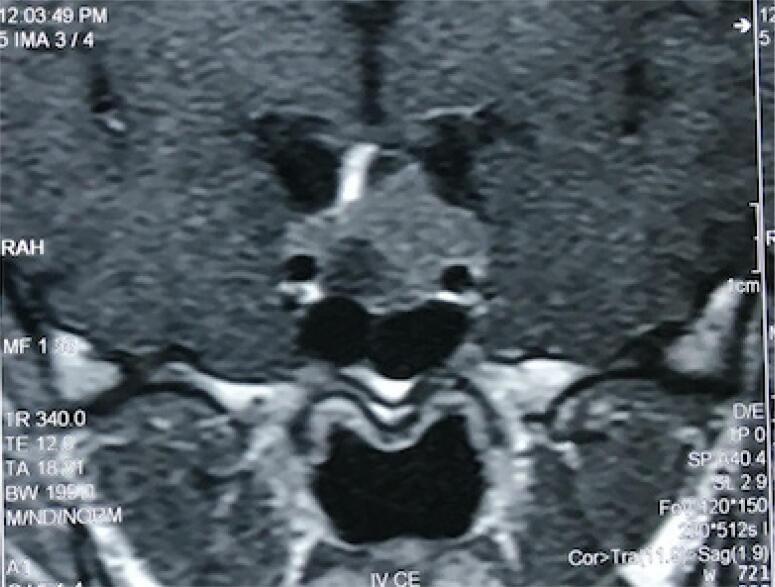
Pituitary MRI revealed a macroadenoma.
